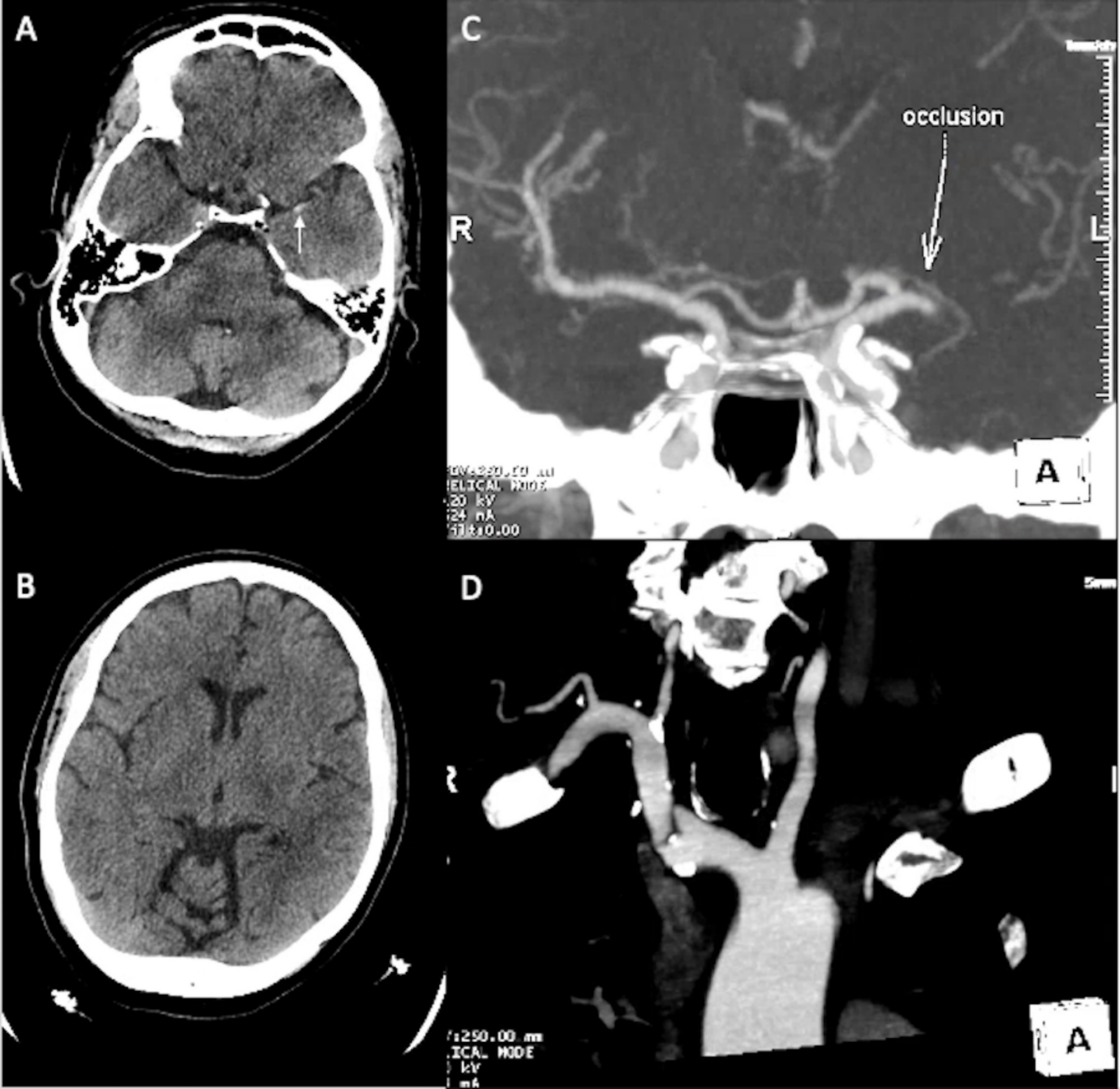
Case 1: neurological imaging. (A) CT of the head shows a left MCA hyperdense sign (white arrow). (B) CT of the head shows no wide extension of left MCA territory infarct. (C) CTA of the head shows the location of MCA occlusion (white arrow). (D) Aortic arch and vessel tortuosity. MCA, middle cerebral artery; CTA, CT angiography
